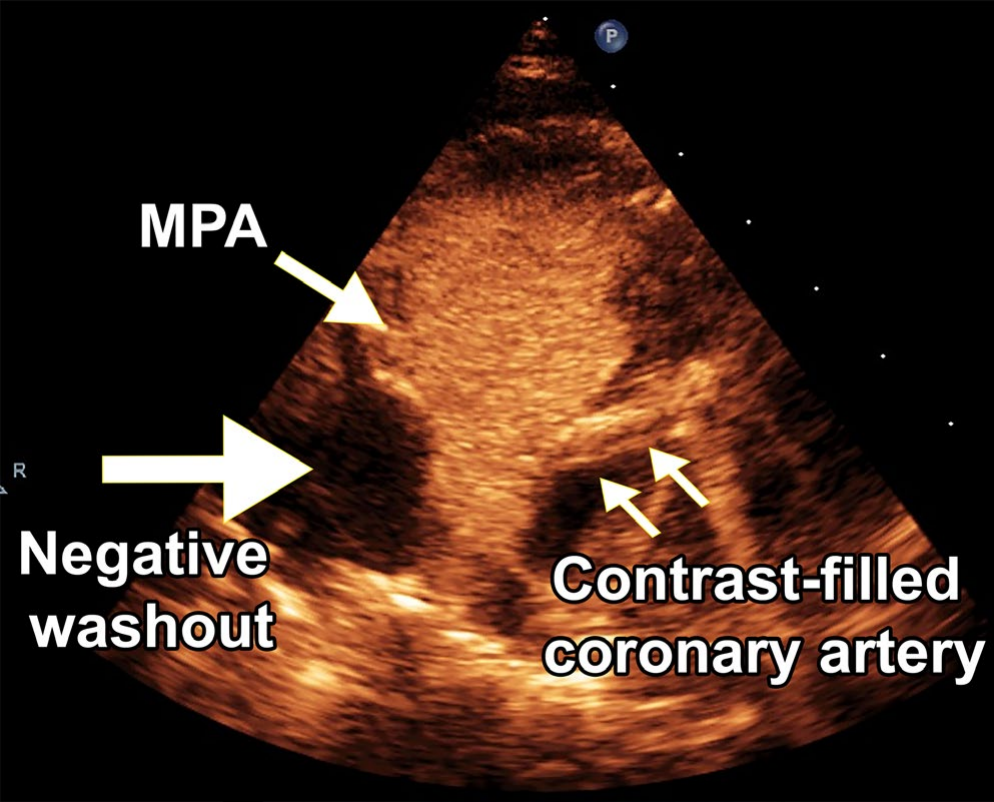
Pulmonary contrast echocardiography. Indicates main pulmonary artery filled with microbubbles in the parasternal short‐axis view (color map is turned on for better visualization). The anomalous left coronary artery is filled with microbubbles (the two smaller white arrows), and the negative washout is also visible at the site of origin of the anomalous LCA, near the origin of the right pulmonary artery (the large white arrow on the left of the Figure). MPA = main pulmonary artery
